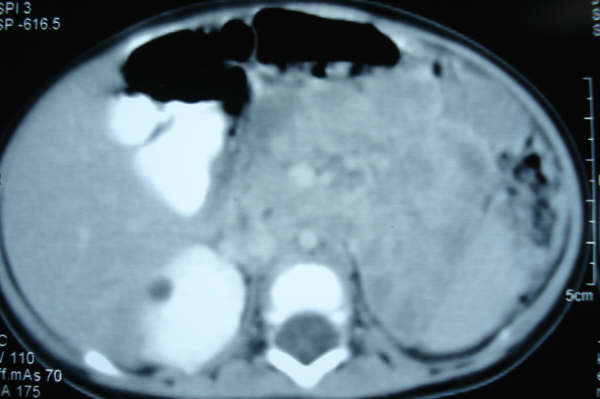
CT Scan showing absence of left kidney.
